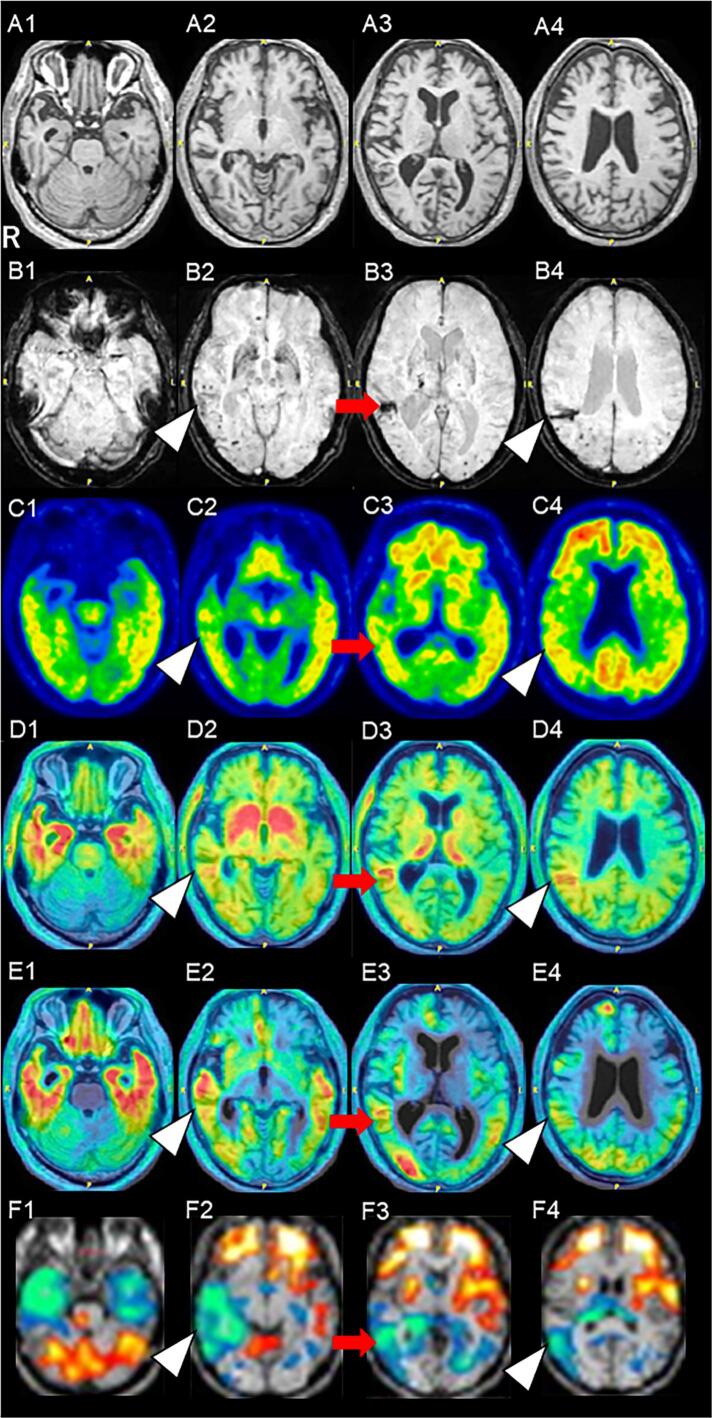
Neuroimaging. T1-weighted MRI (T1WI) (X/04). B. SWI MRI (X + 2/04). ^11^C-PiB-PET (X/07). D. ^18^F-THK5351-PET (X + 2/11) superimposed on T1WI MRI. E. ^18^F-MK-6240-PET (X + 9/10) superimposed on MRI T1WI. F. ^99m^Tc ECD-SPECT (eZIS) (X + 9/02). Sections shown A1–F4: transverse view.
